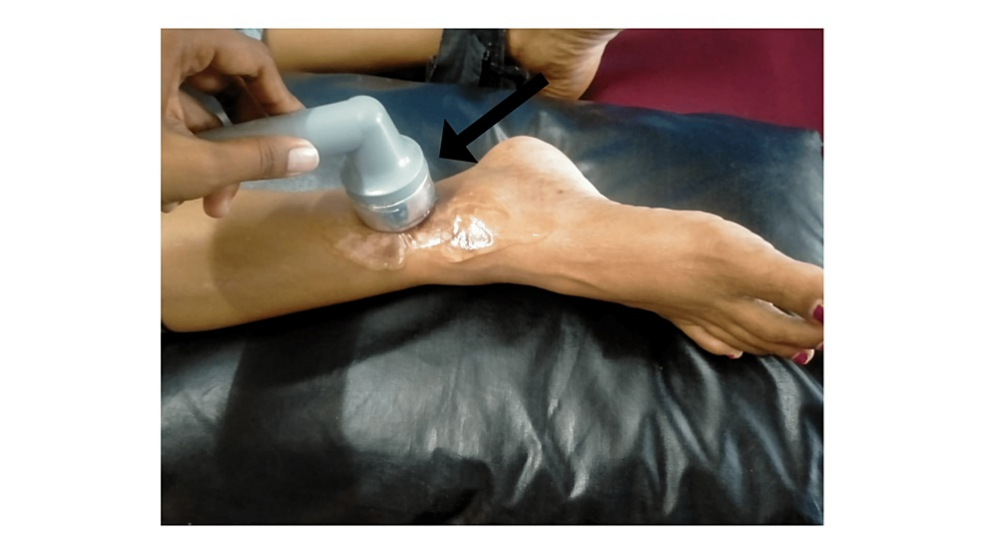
Scar being treated with ultrasound
